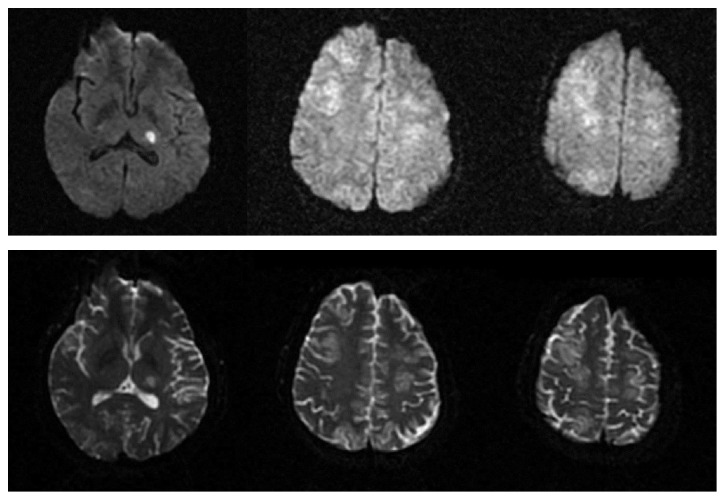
Case No. 1. DWI sequences (upper images) and ADC maps (lower images) showing diffusion restriction in left thalamus and mild signal hyperintensity in the same areas described in Figure 1.
